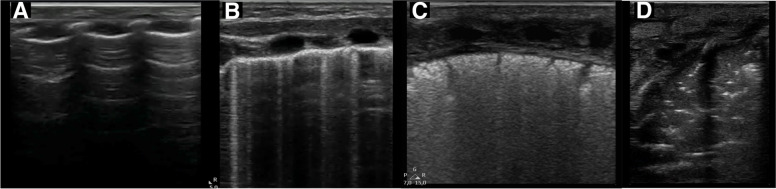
Lung ultrasound area score. Lung ultrasound score for each area is attributed according to the following criteria: 0, A-pattern (defined by the presence of only A-lines; panel A); 1, B-pattern (defined as the presence of three or more B-lines, well spaced; panel B); 2, severe B-pattern (defined as the presence of crowded and coalescent B lines with or without consolidations limited to subpleural space; panel C); and 3, extended consolidation (panel D)
